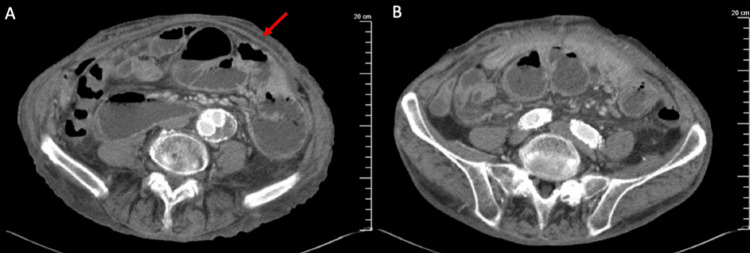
Preoperative abdominal CT (A) Peritoneal thickening (red arrow); (B) thickened subfascial plane CT, computed tomography
